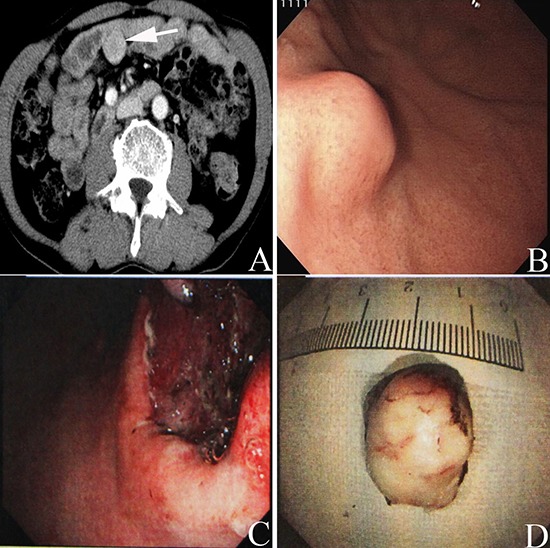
Preoperative abdominal enhanced CT scan showed a mass (white arrow in A) with a size of 3.5cm×3 cm located in the abdominal cavity in one patient with rectal cancer Upper gastrointestinal endoscopy presented an intramural lesion (B) located in the body of the patient with GIST of the stomach synchronous with a small carcinelcosis located in the antrum of the stomach. Tumor complete resection was obtained through the endoscopic view (C) Image of the tumor resected through endoscopic resection; the tumor size was lower than 2 cm (D).
